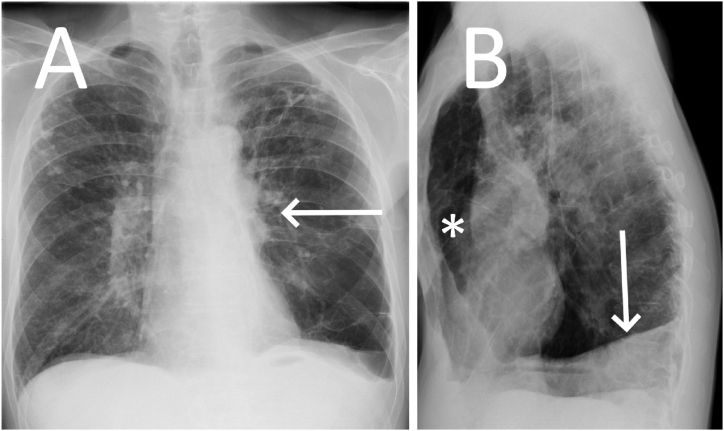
Posteroanterior (A) and lateral (B) chest X-rays demonstrating a small left hilum (A, arrow) when compared to the right side. The lateral chest X-ray shows a loss of lung volume with the ascension of the left hemidiaphragm (B, arrow) and right lung herniation into the anterior mediastinum (B, star).
